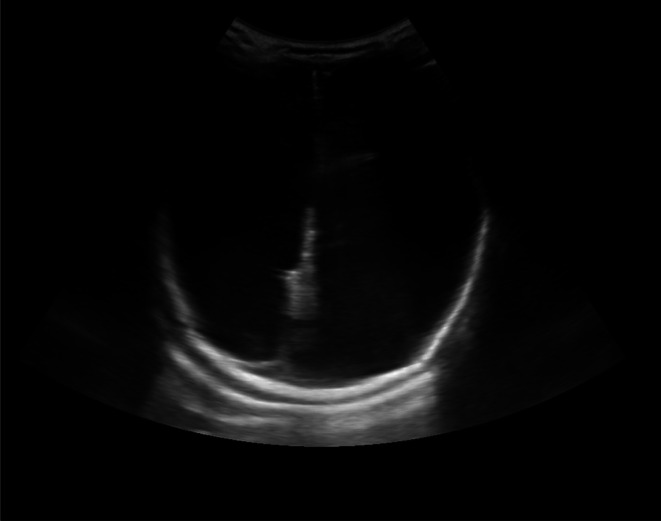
Ultrasound cranium axial view showing dilated ventricle falx is visualized.
